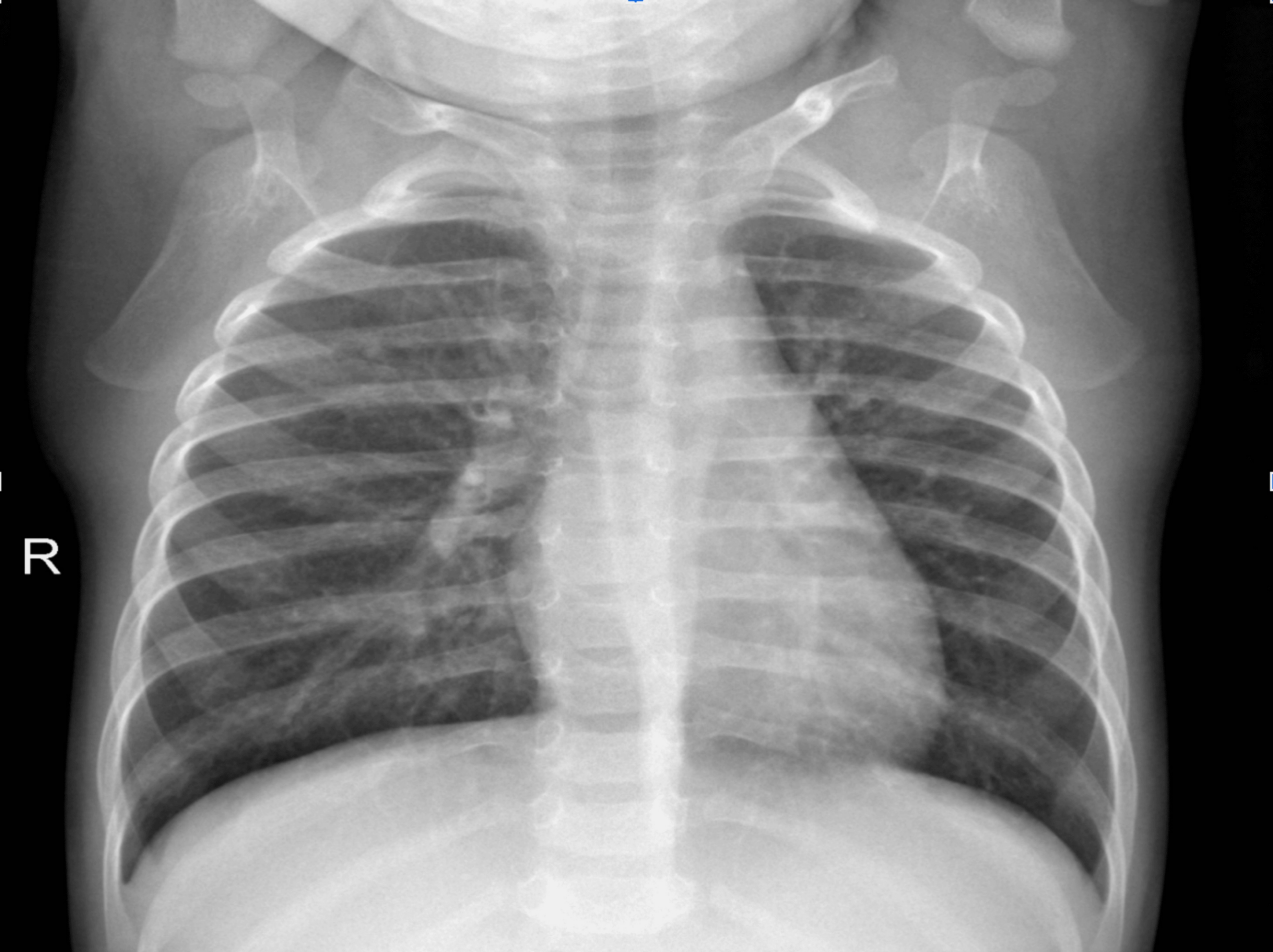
Normal chest X-ray. An example of the normal chest X-ray used to train our model. Chest X-ray images. Accessed on January 5, 2024, from https://www.kaggle.com/datasets/tolgadincer/labeled-chest-xray-images.
